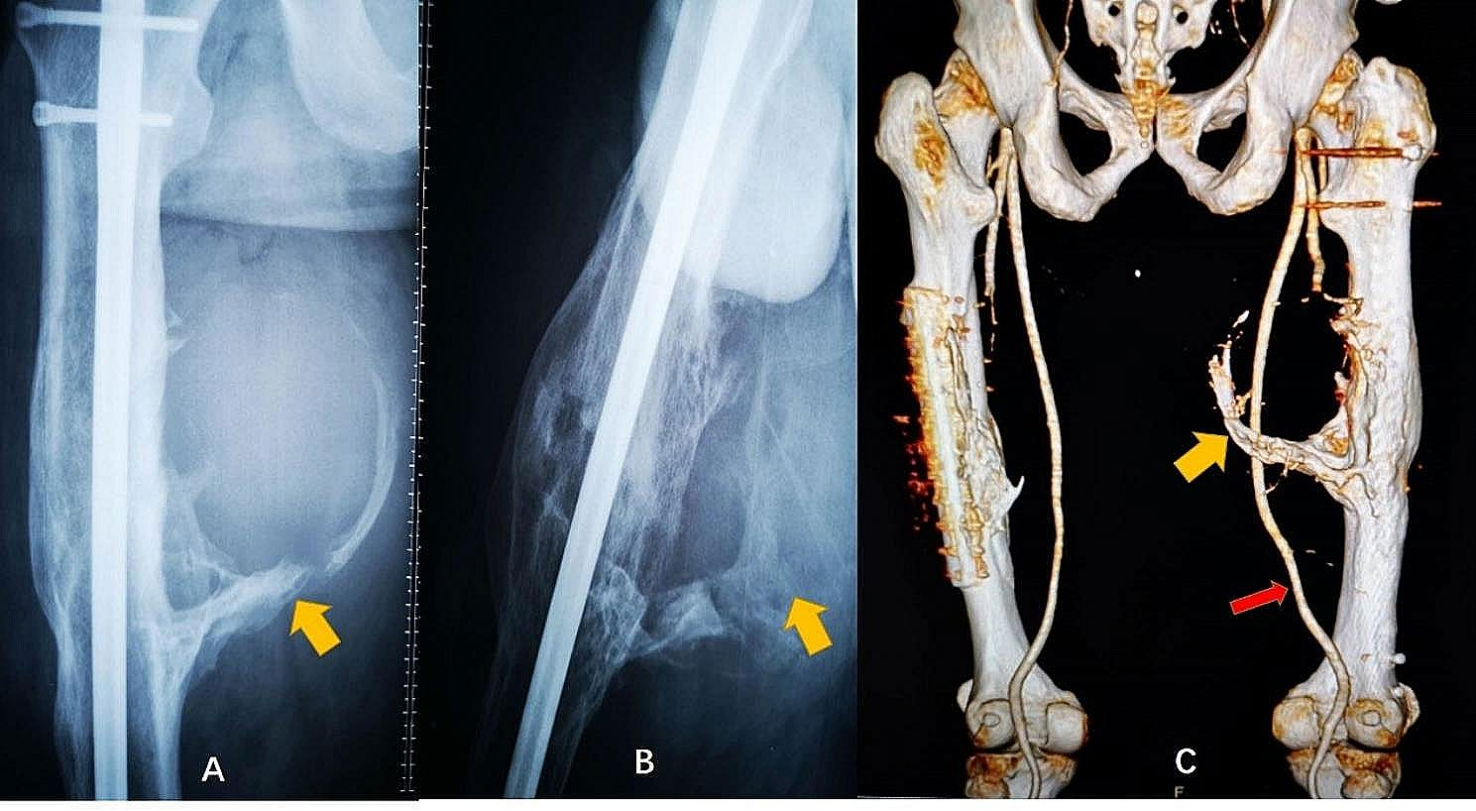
Preoperative radiographs. Anteroposterior (A), lateral radiographs (B), and three-dimensional CTA images (C) demonstrating severe heterotopic ossification (yellow arrow) in the medial-posterior part of the right thigh. The femoral profound artery (red arrow) is seen nearby the heterotopic ossification
